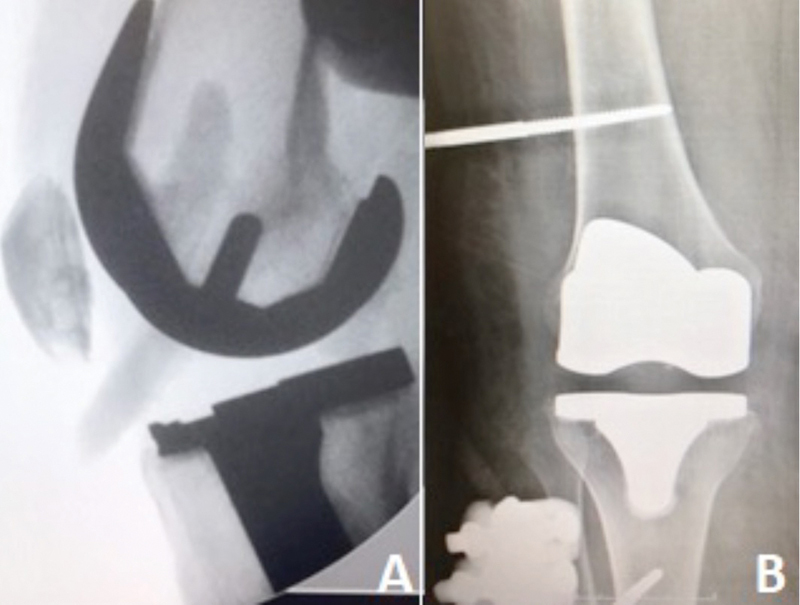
Lateral post reduction radiographic assessment (
